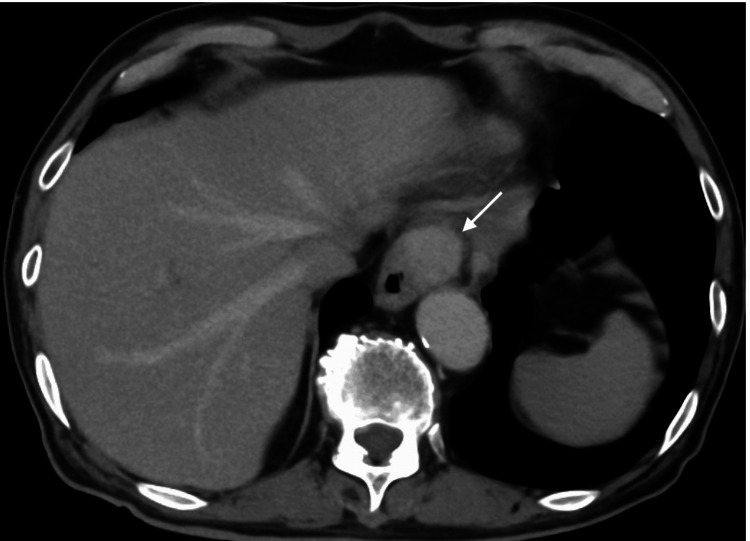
CT findings CT showed an enhanced mass (arrow) at the cardia. CT: computed tomography
